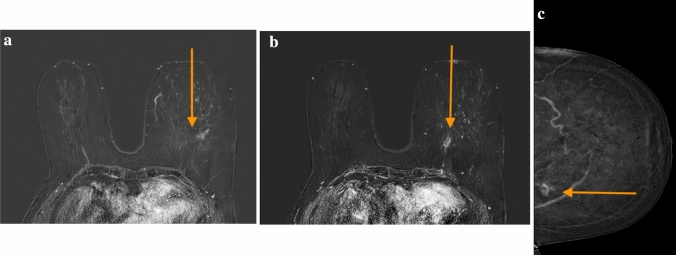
a Discordance in patient 2 MRI, left side first lesion [1]. b Discordance in patient 2 MRI, left side second lesion [2]. c Discordance in patient 2 CEM, left side unique lesion
